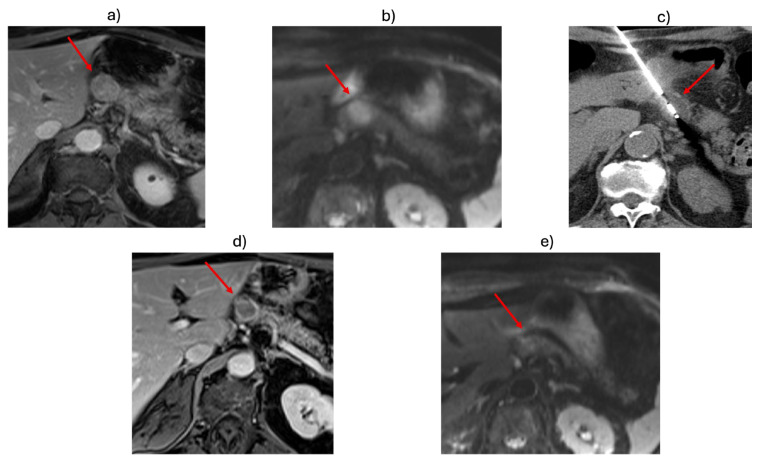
Representative case of transgastric CT-guided cryoablation for pancreatic head adenocarcinoma. A representative case of painful unresectable pancreatic head adenocarcinoma treated with percutaneous CT-guided cryoablation through a transgastric approach. (a) Pre-procedural contrast-enhanced CT showing the pancreatic head lesion. (b) Pre-procedural MRI with diffusion-weighted imaging (DWI) confirming the lesion. (c) Intraprocedural CT image obtained during transgastric cryoablation. (d) Contrast-enhanced CT at 12-month follow-up. (e) Follow-up DWI-MRI at 12 months showing no appreciable residual diffusion restriction at the treated site. Arrows indicate the target lesion, cryoprobe position, and corresponding treated area during follow-up. Follow-up imaging demonstrates sustained reduction in the treated lesion, consistent with a local cytoreductive effect after cryoablation.
